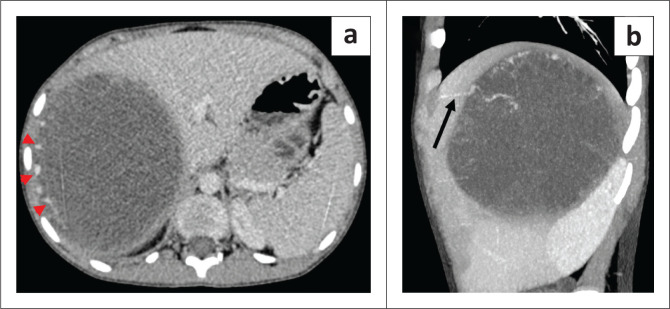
(a) Axial contrast-enhanced CT images demonstrating the cystic appearance of the mass with eccentric internal vascularity (red arrow heads); (b) Sagittal maximum intensity projection image showing a tortuous artery supplying the periphery of the lesion as denoted by the black arrow.
